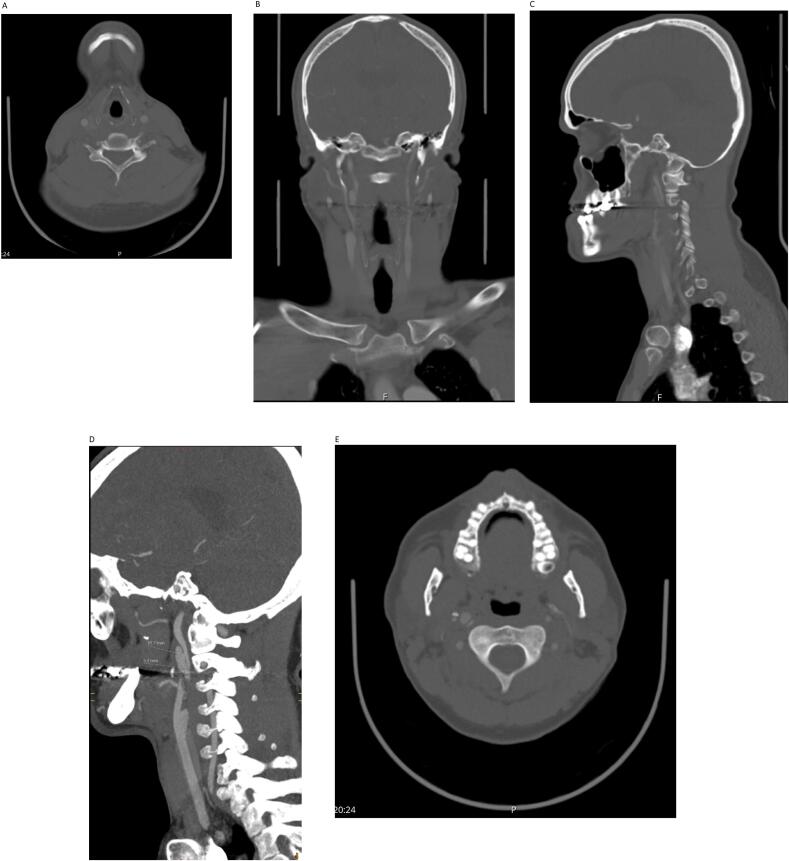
A: CT angiography, axial view showing no dissection in both common carotid arteries. B: CT angiography with coronal reconstruction showing right ICA pseudoaneurysm. C: CT angiography with sagittal reconstruction showing right ICA pseudoaneurysm. D: CT angiography with sagittal view showing right ICA pseudoaneurysm (15.2 × 5.3 mm). E: Axial cut showing dissection in the right ICA.
